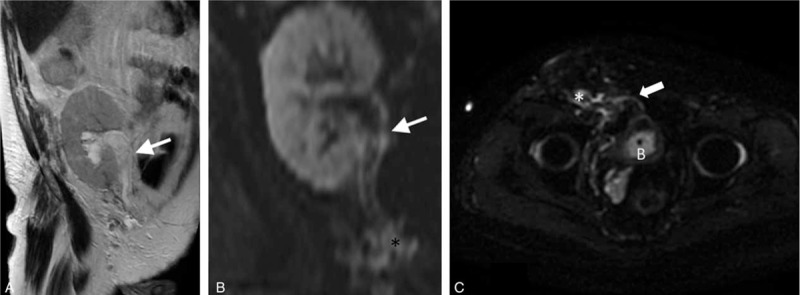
Magnetic resonance imaging performed 2 days after reoperation demonstrates mild, residual hydronephrosis and minor fluid collection (∗) at the site of previous plug positioning, near the passage of the ureter (arrow). B: bladder.
